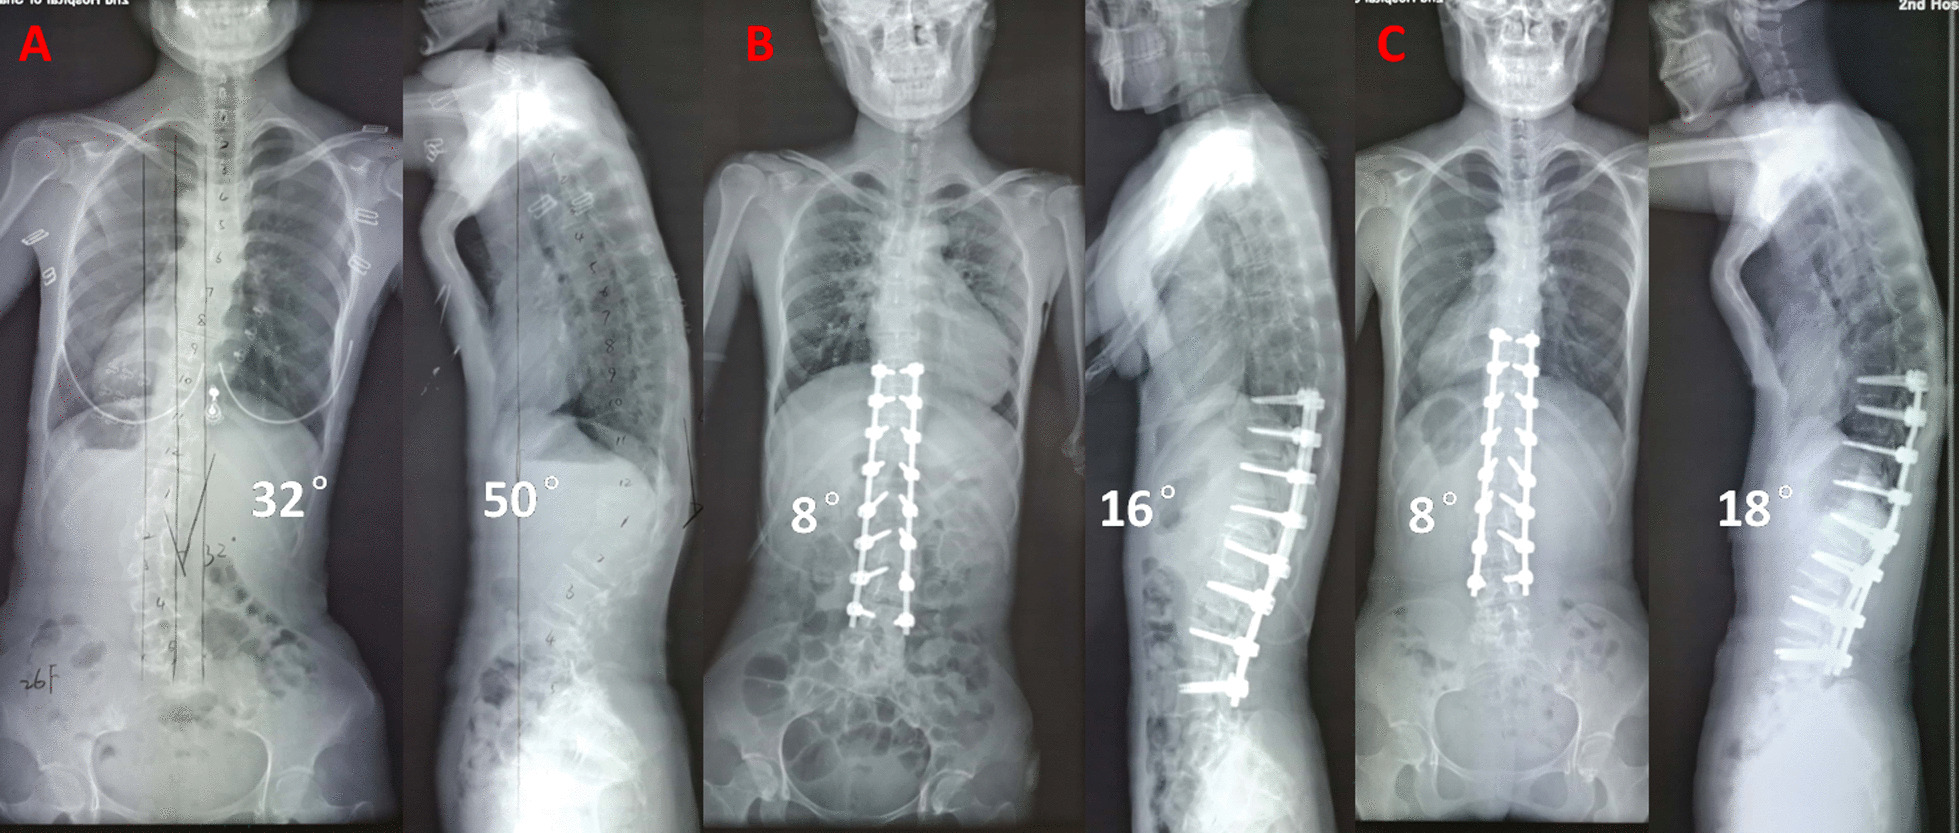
A 26-year-old female patient with thoracolumbar spinal stenosis, kyphoscoliosis and pectus excavatum. She underwent extensive laminectomy, ponte osteotomy and posterior fusion surgery (T9-L4). The kpphoscoliosis was improved significantly in preoperative and postoperative radiographs (A,B). At 6 months follow-up, the curve correction and spinal alignment were maintained very well(C)
